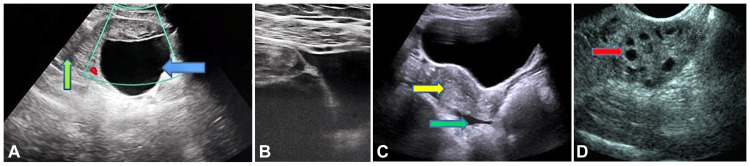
(A) A gray-scale B-mode ultrasound image revealed a bulky right ovary with peripherally arranged follicles measuring approximately 8.1 x 6.1 cm. A well-defined anechoic cystic lesion, measuring approximately 6.7 x 4.1 cm, was noted in the right ovary, with a thickened surrounding stroma appearing hypoechoic due to edema. The lesion showed no vascularity on color Doppler (blue arrow). There was evidence of minimal pericystic fluid collection and surrounding fat stranding (green arrow). These features are suggestive of complete ovarian torsion. (B) A gray-scale B-mode ultrasound image showed mild free fluid in the pelvis (white arrow). (C) A gray-scale B-mode ultrasound image depicted a normal uterus (yellow arrow) and mild fluid collection in the pouch of Douglas (green arrow). (D) A gray-scale B-mode ultrasound image of the left ovary showed a normal appearance with multiple follicles (red arrow).
